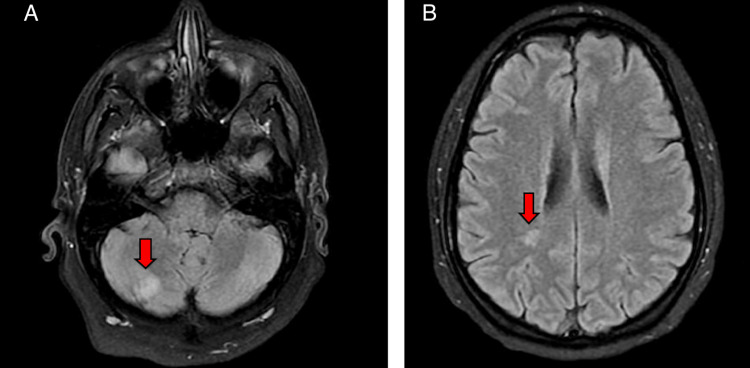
A and B demonstrate multiple acute infarcts throughout the supra- and infratentorial brain and brainstem noted on MRI of the head (red arrows). MRI: magnetic resonance imaging
